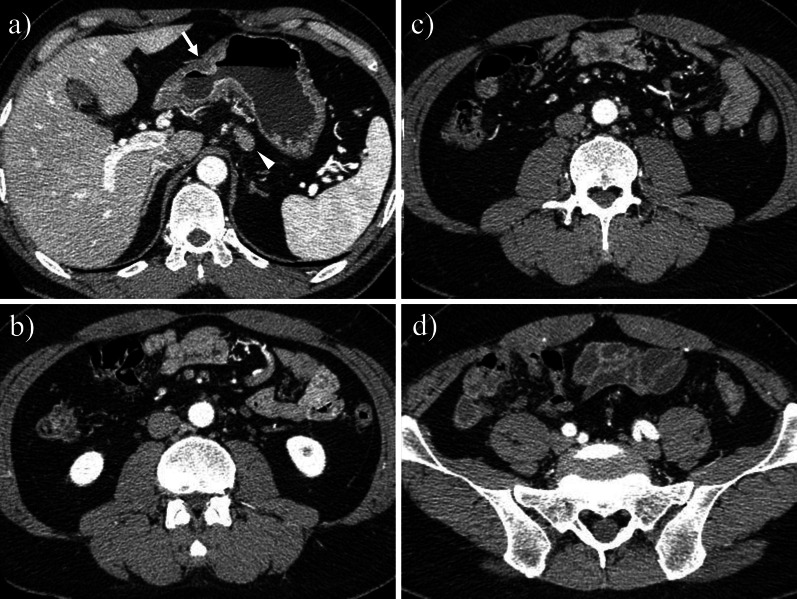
Preoperative CT. a CT revealed wall thickening with enhancement of the gastric angle (arrow) and regional lymph node swelling (arrowhead). b–d No obvious bone metastasis was observed
